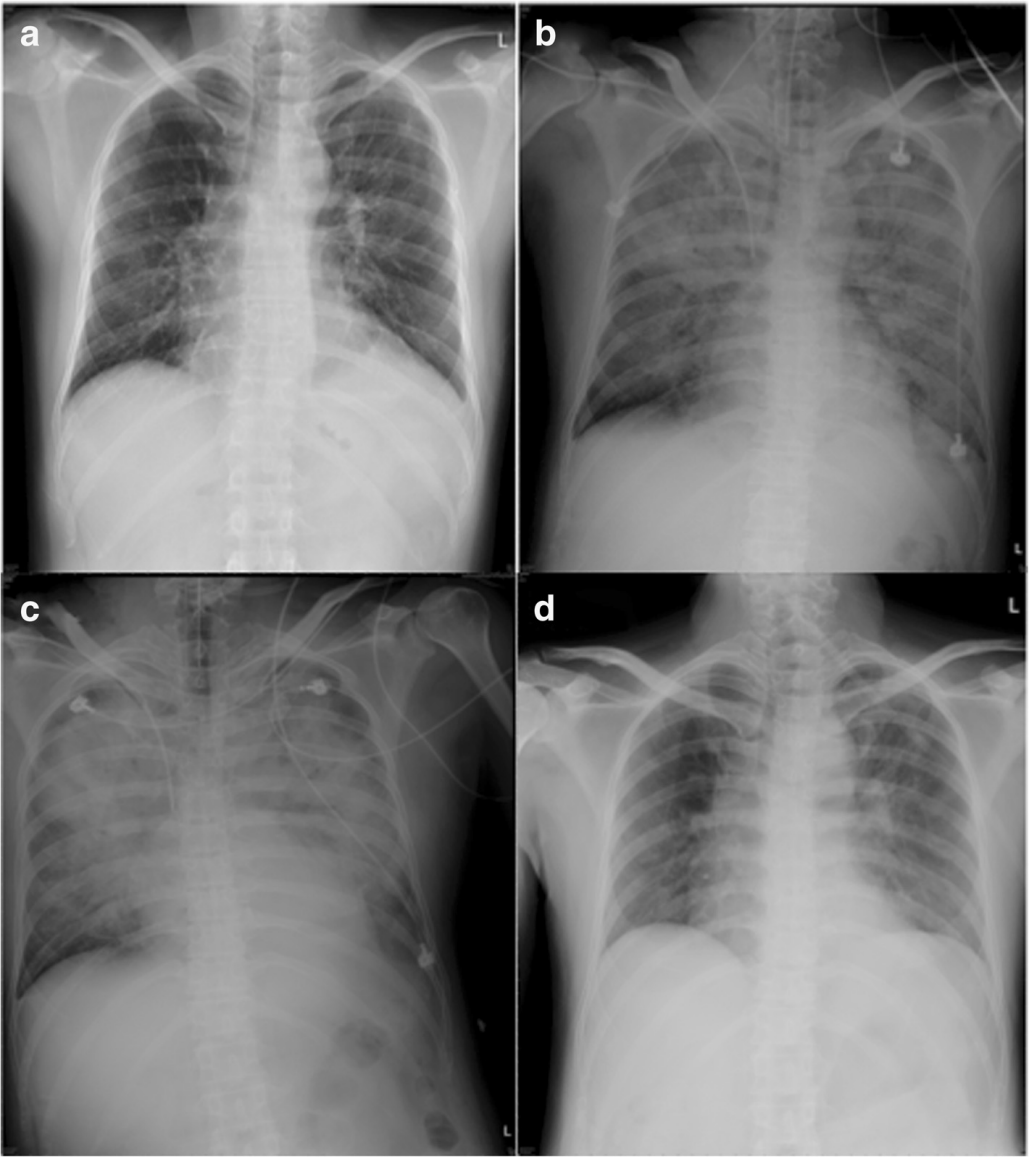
Initial chest radiography demonstrated mild pulmonary infiltration over the left lung on day 1 (a). A portable chest X-ray revealed prominent bilateral infiltrates at 48 h later after admission to our hospital (b), progressed to worse picture on day 4 (c) and recovery to near-normal x ray on day 22 (d)
